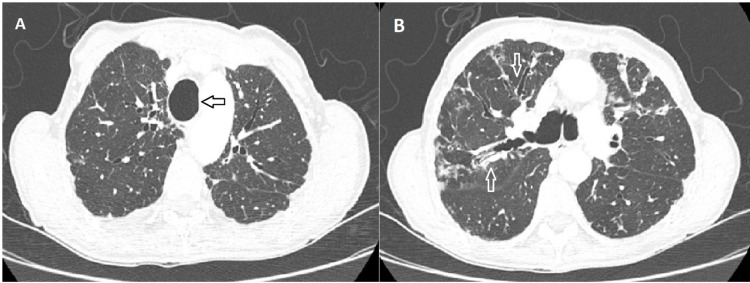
Axial HRCT images. (A) Axial HRCT showing a large tracheal size. (B) Axial HRCT showing bronchiectasis with dilated bronchi (arrows). HRCT: high resolution computed tomography
